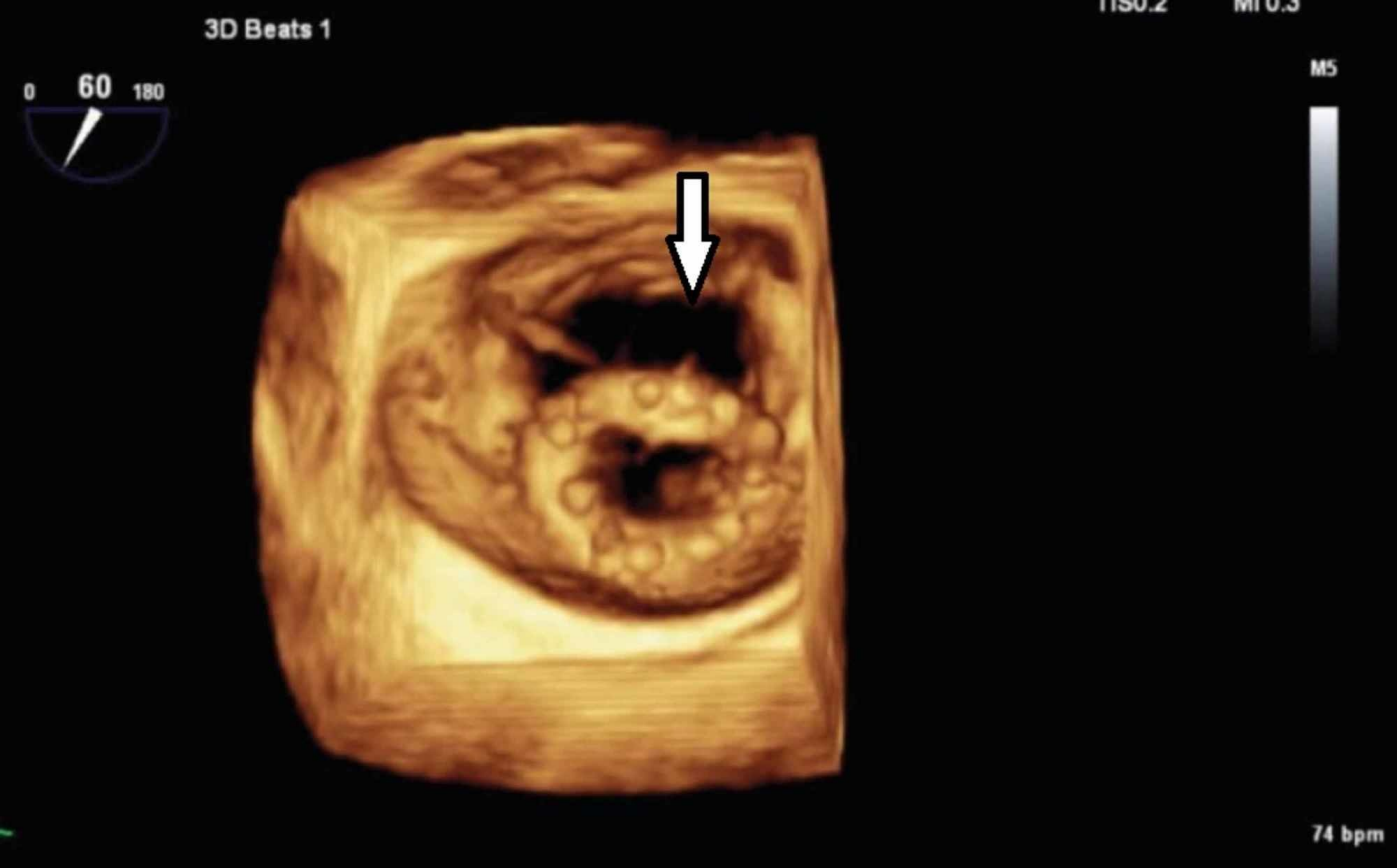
3D TEE imaging without color revealing significant ring dehiscence (white arrow) along the anterior mitral valve. TEE, transesophageal echocardiogram
